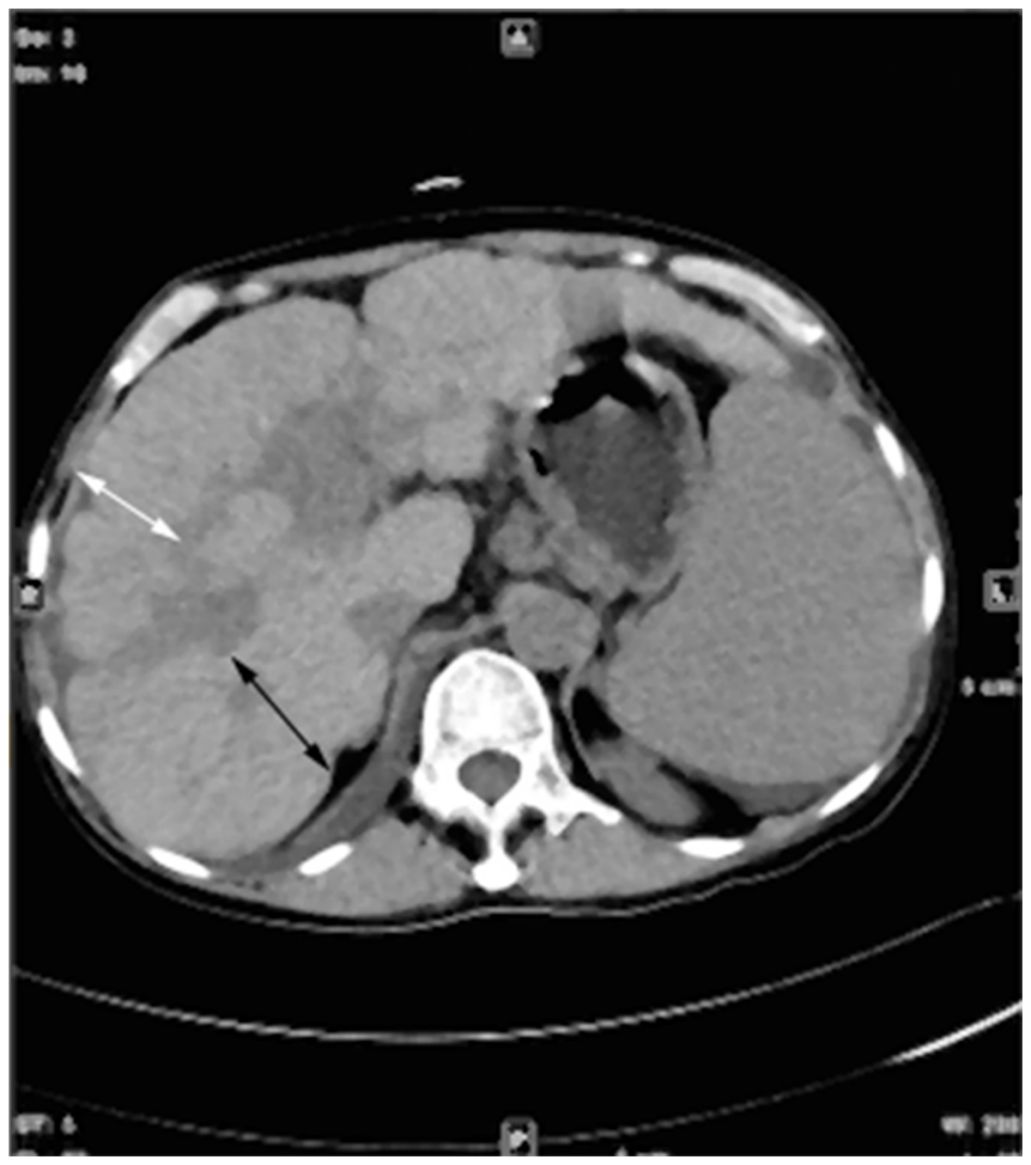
Cirrhotic morphology in CT imaging. Portal venous phase CT scan shows nodularity on the liver surface and heterogeneity of the liver parenchyma. A ratio of transverse caudate lobe width (black arrow) to right lobe width (white arrow) is greater than 0.65. Splenomegaly was present.
